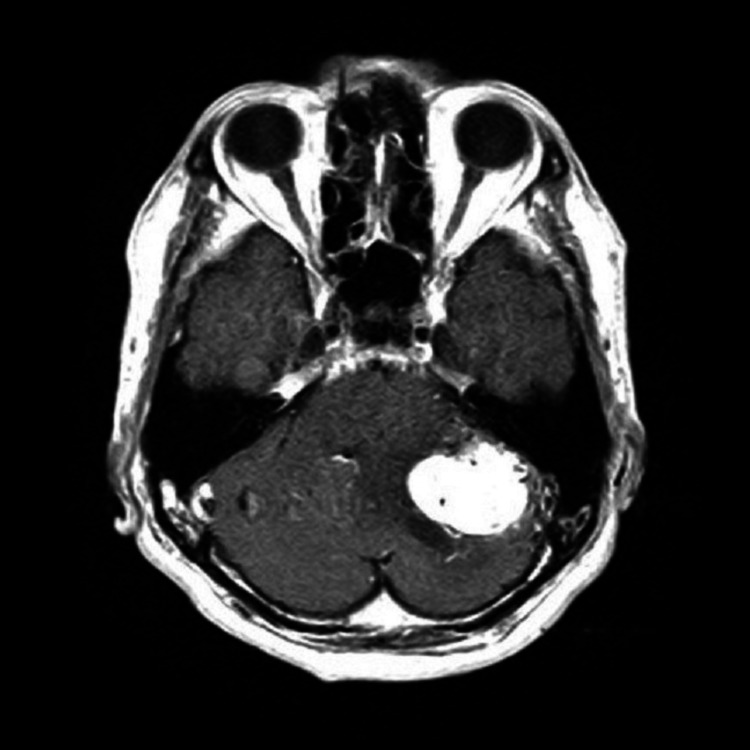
Magnetic resonance imaging (MRI) on admission. Gadolinium (Gd)-enhanced T1-weighted  MRI showed a large tumor in the left cerebello-pontine angle.
